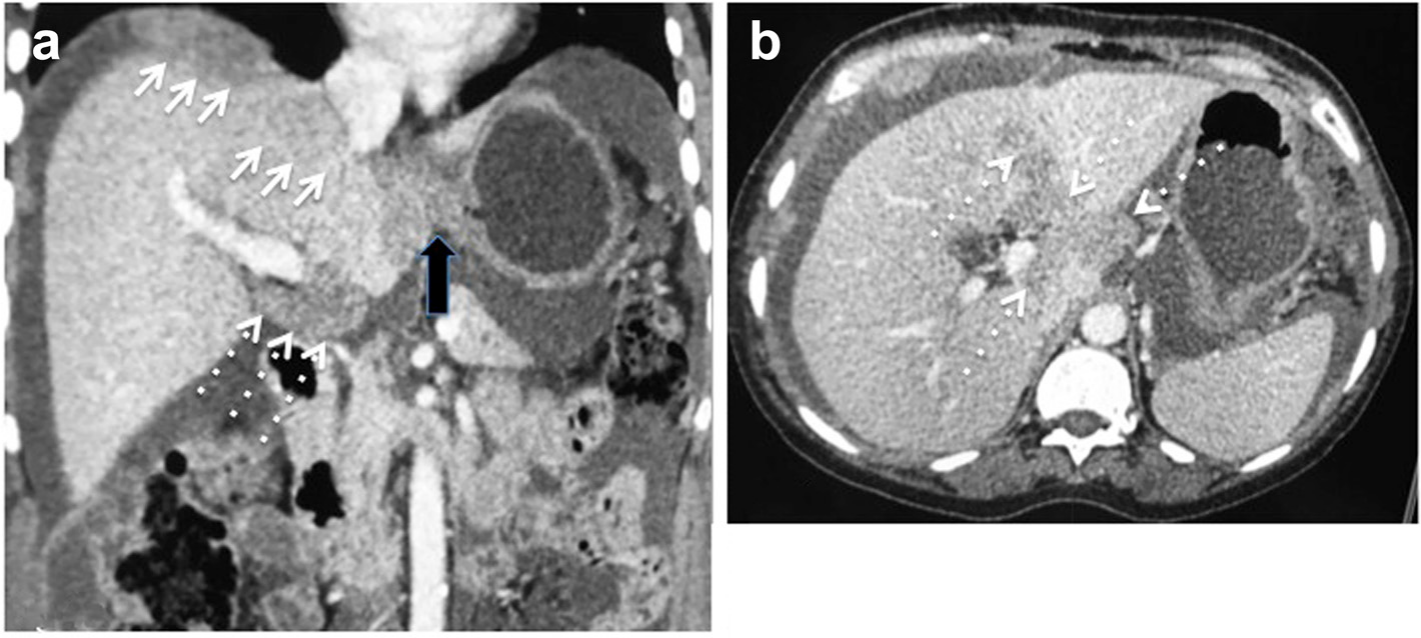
a and b 64 year old woman with stage IIIC clear cell carcinoma of the ovary. Axial and Coronal reconstructed CT demonstrating non resectable dense confluent right subdiaphragmatic disease (arrows), diffuse thick plaque disease in the gastro-hepatic ligament (block arrow) and an deposit >2 cm in the hepato-duodenal ligament and porta hepatis (dashed arrows)
